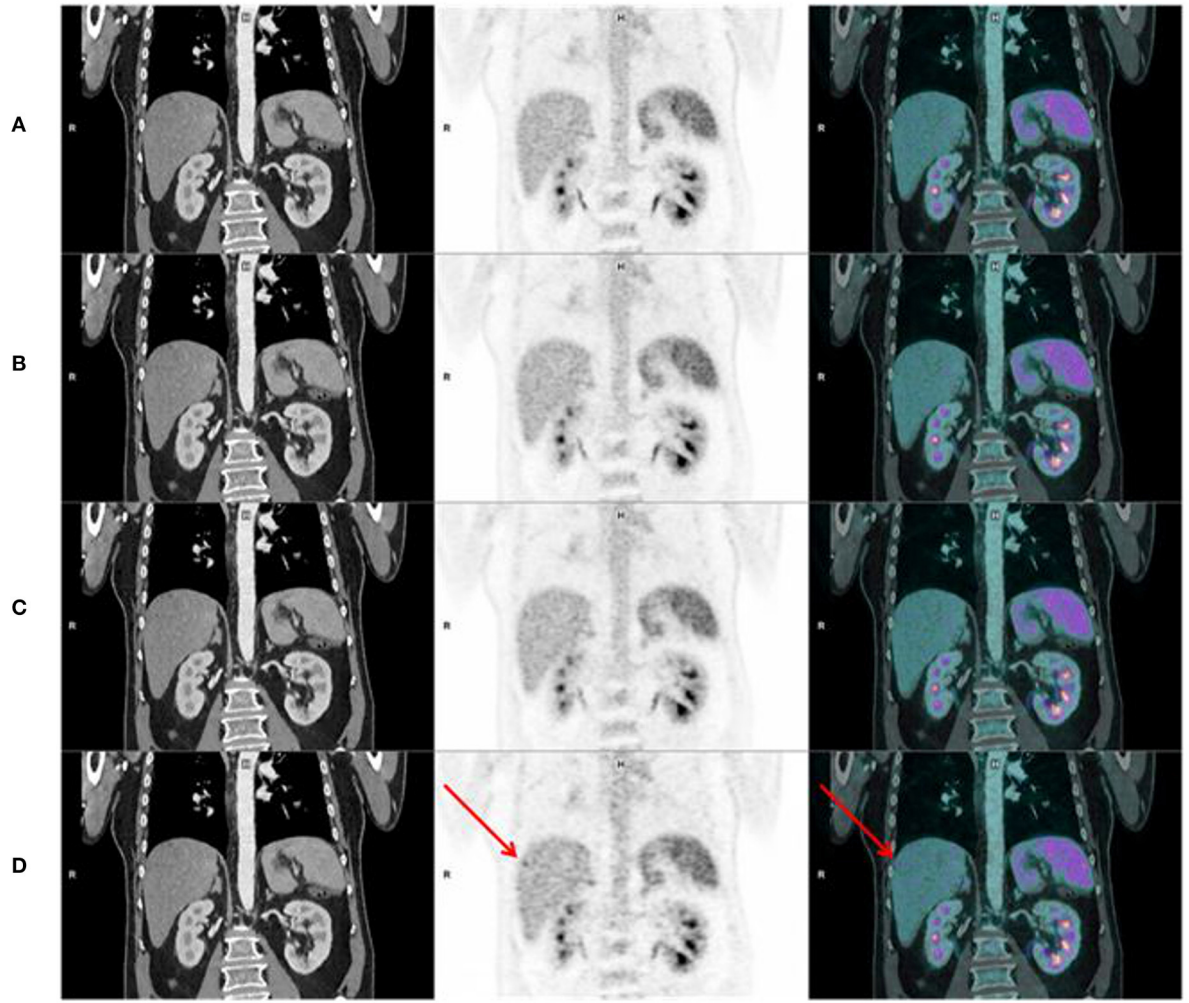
Coronal CT, PET, and fusion images in the same patient, showing better sharpness of organs, pyelo-ureteral uptakes, and less hepatic noise when increasing acquisition time [(A-D) from top to bottom, respectively, 120, 90, 60, and 30 s per bed position]. We can see noise on the “Analog-like” acquisition which can mimic focal uptake in the right liver (arrow), and which disappears on the other acquisitions.
